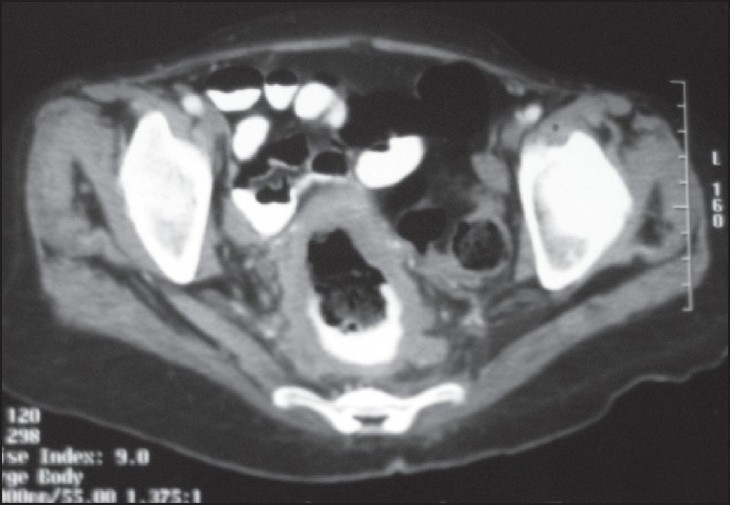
CT scan of the abdomen shows rectal mass with perirectal infiltration
